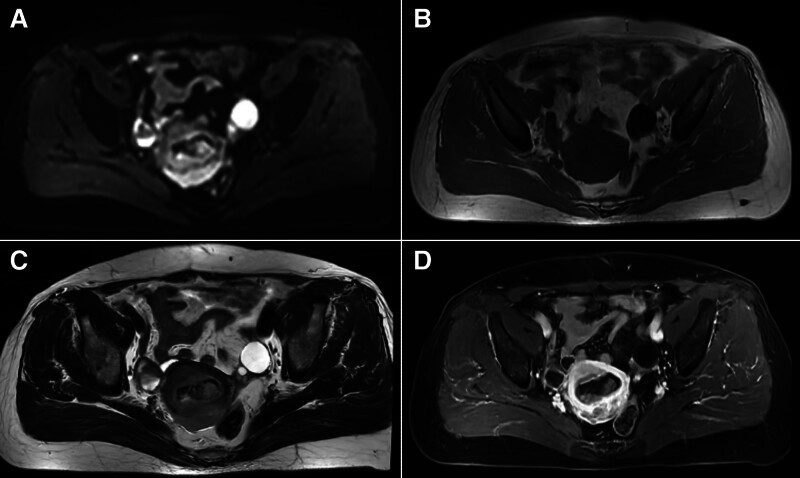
(A–D) Abdominal magnetic resonance imaging (MRI) shows that the uterus is slightly enlarged, with irregular morphology, uneven signal in the uterine cavity, and multiple patchy soft tissue nodules. DWI shows an uneven high signal, T1WI shows isolow signal, T2WI shows a mixed high and low signal, with unclear edges. The enhancement scan is uneven, with a degree of enhancement lower than that of the normal uterine muscle layer, and there is a patchy area without enhancement. DWI = diffusion weighted imaging, T1WI = T1-weighted imaging, T2WI = T2-weighted imaging.
